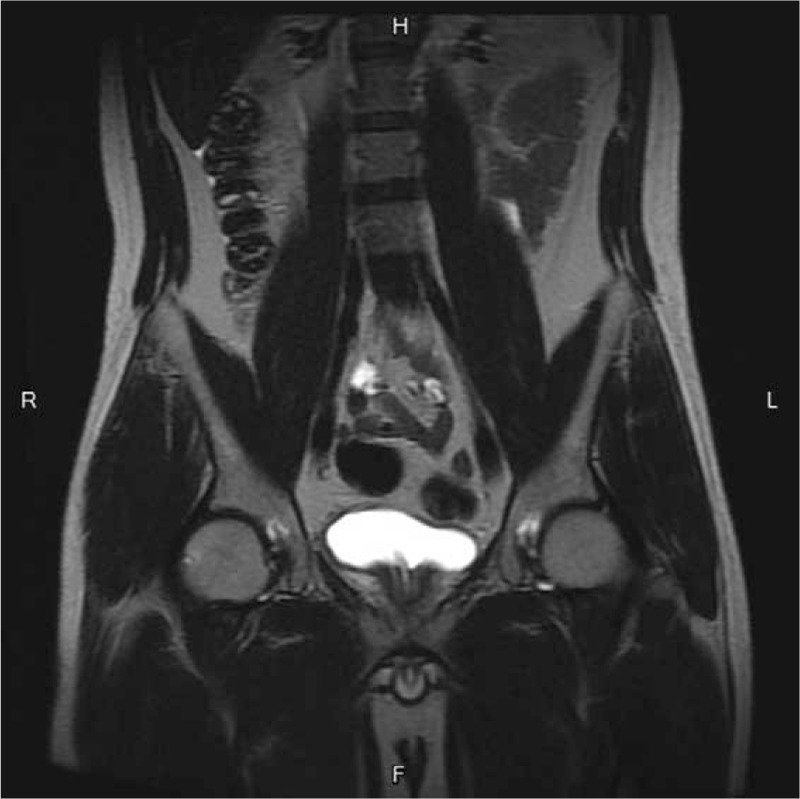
MRI of the bladder showed neither thickening of the bladder wall nor the existence of nodules.
